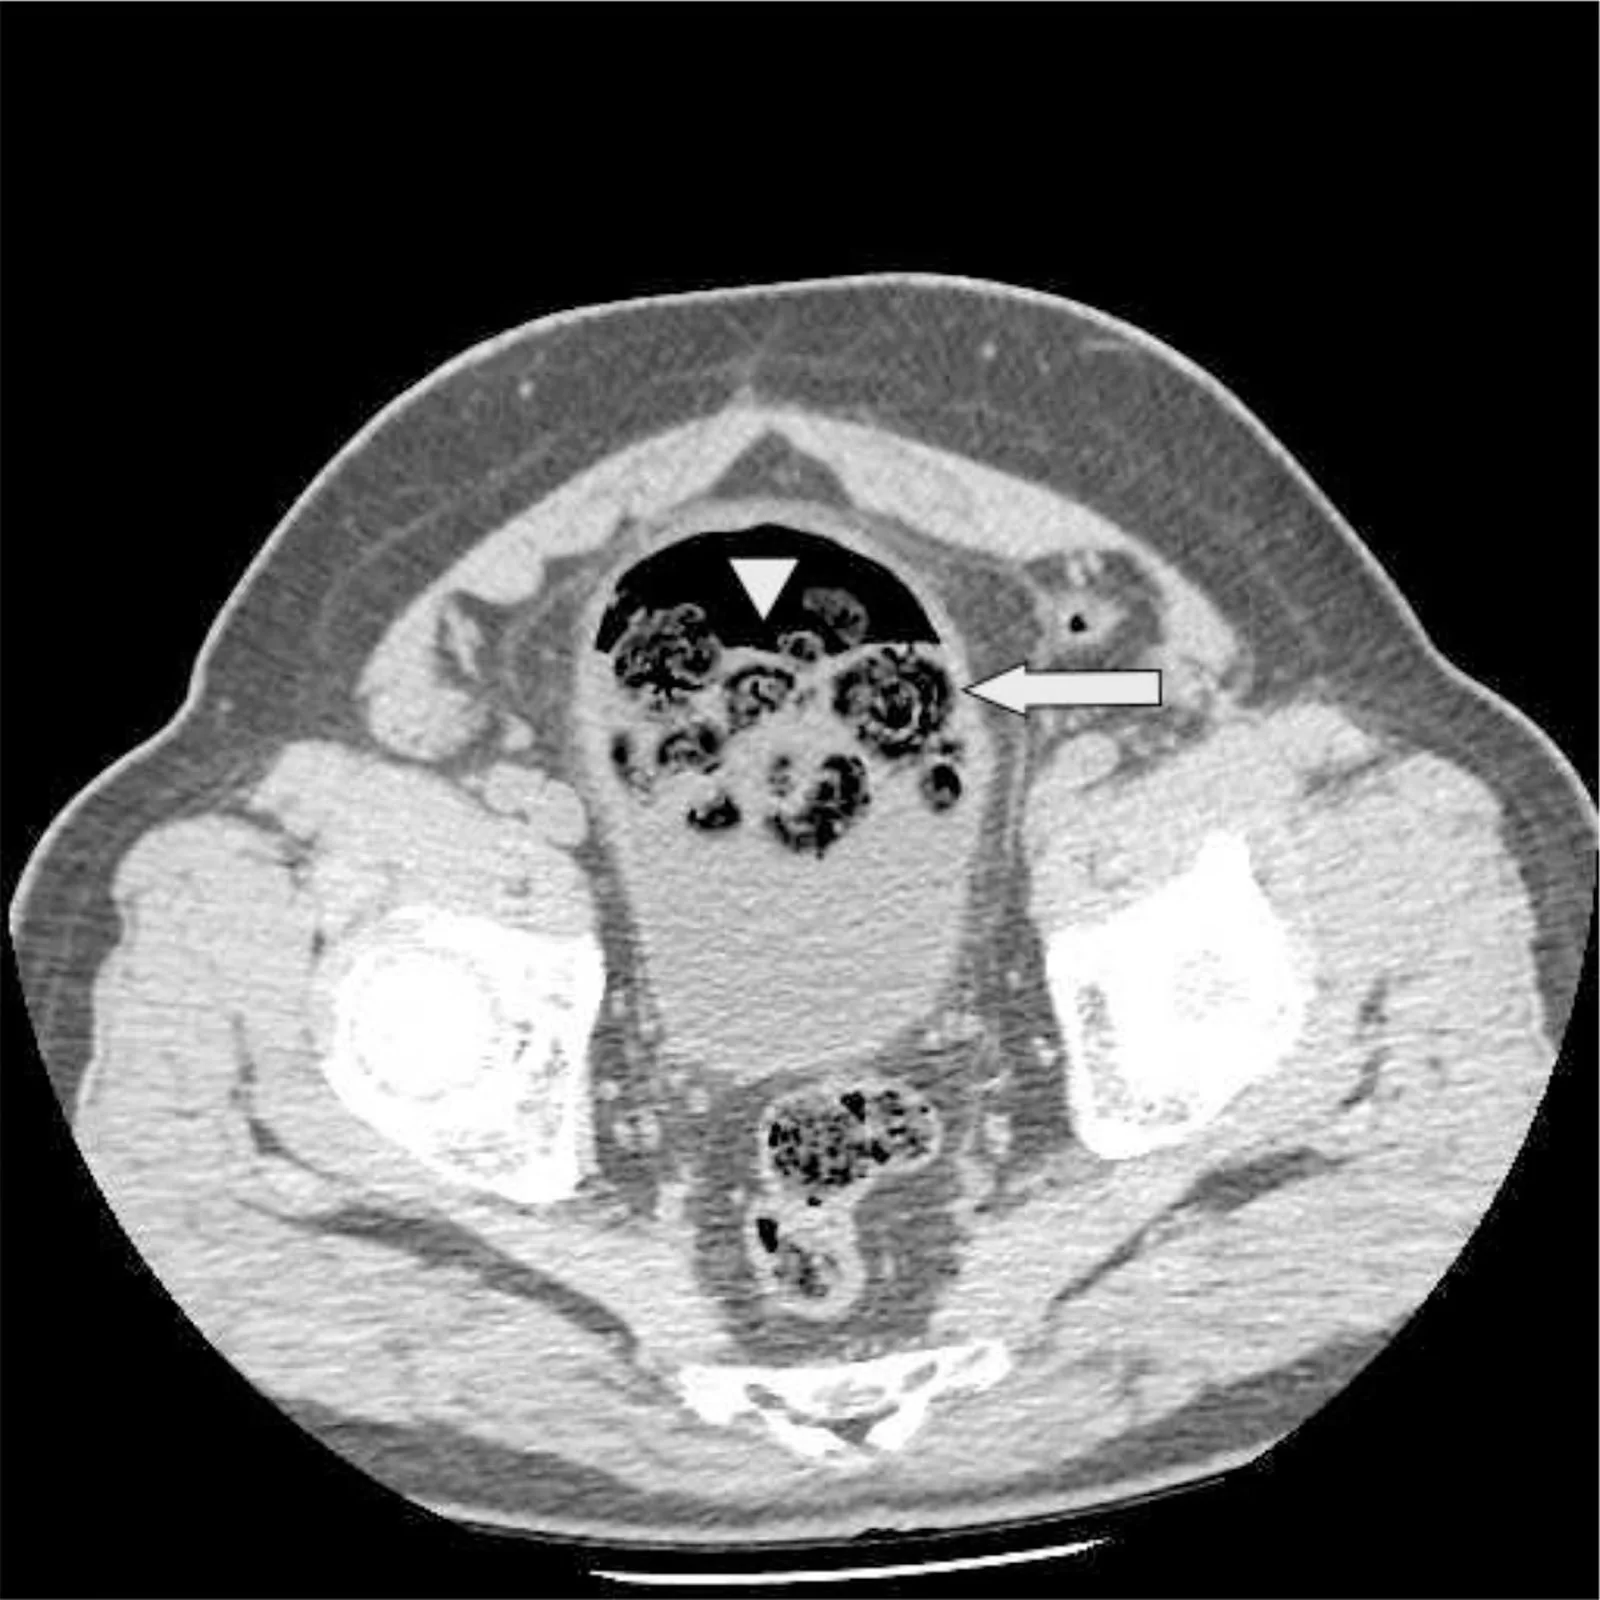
Axial noncontrast abdominal CT scan at admission shows multiple irregular, mixed-attenuation nodules (arrow) within the bladder lumen, associated with intraluminal gas (arrowhead), consistent with fungal balls and emphysematous cystitis.
